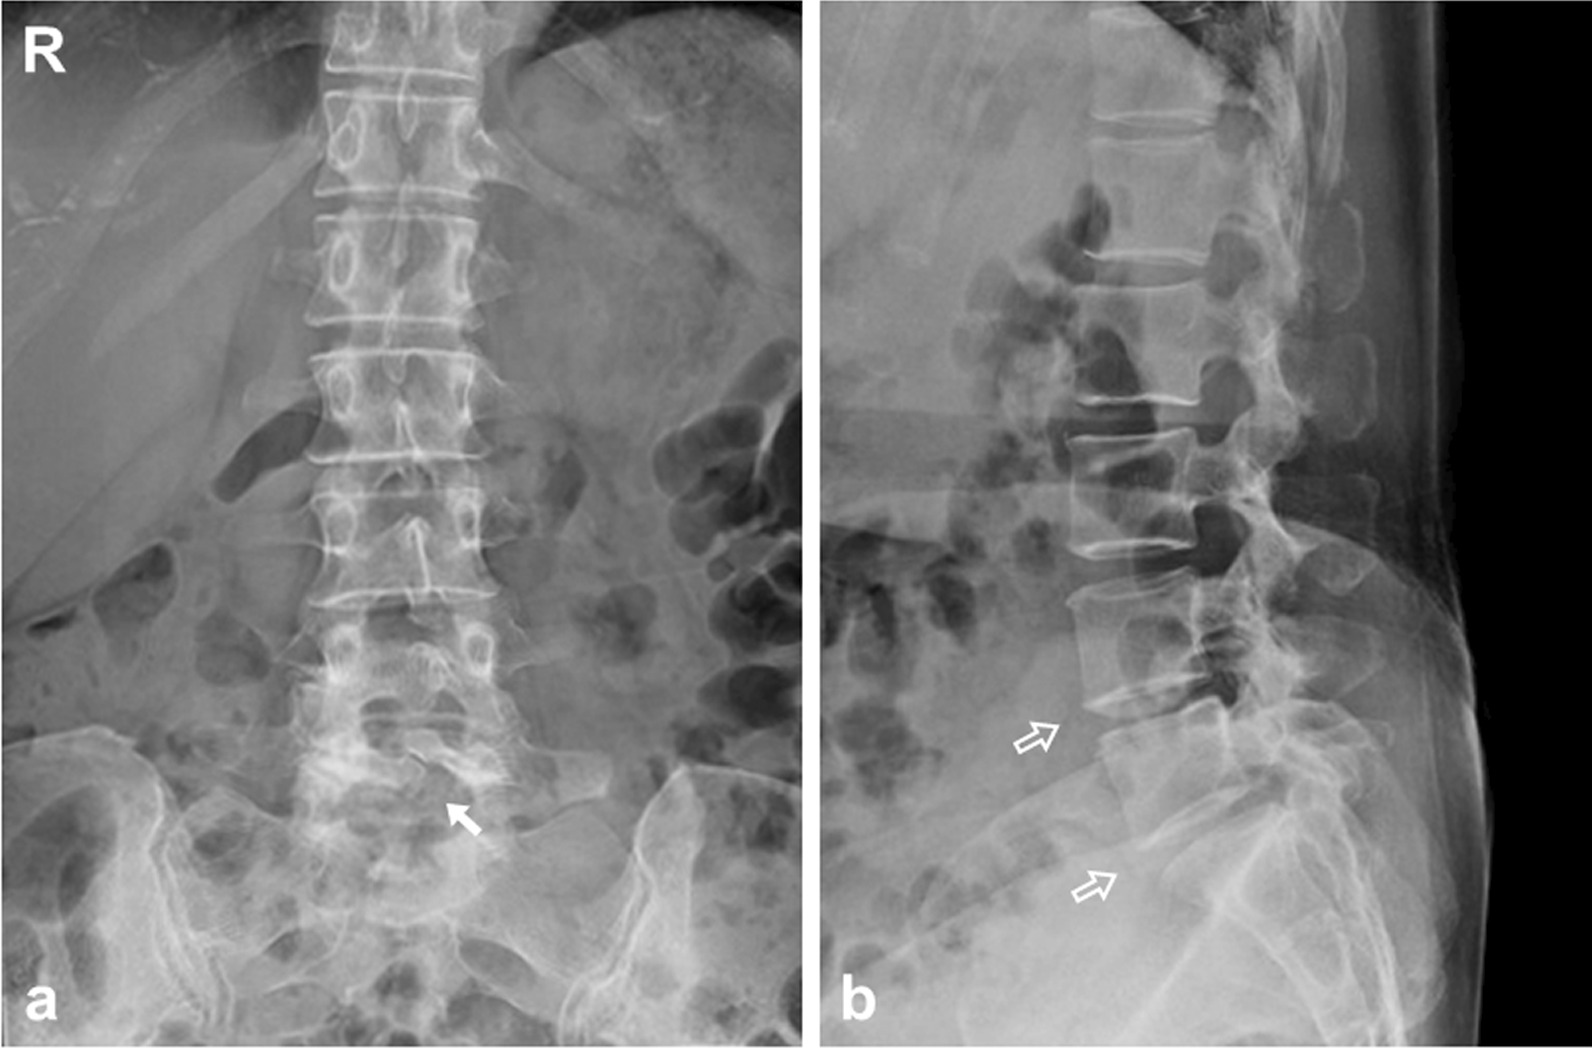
Preoperative plain radiographs. Spina bifida occulta at the L5 neural arch was detected in the anteroposterior direction (white arrow), and slight disc space narrowing at the L4/5 and L5/S intervertebral disc levels was seen in the lateral direction (open arrows). a a-p view. b lateral view.
